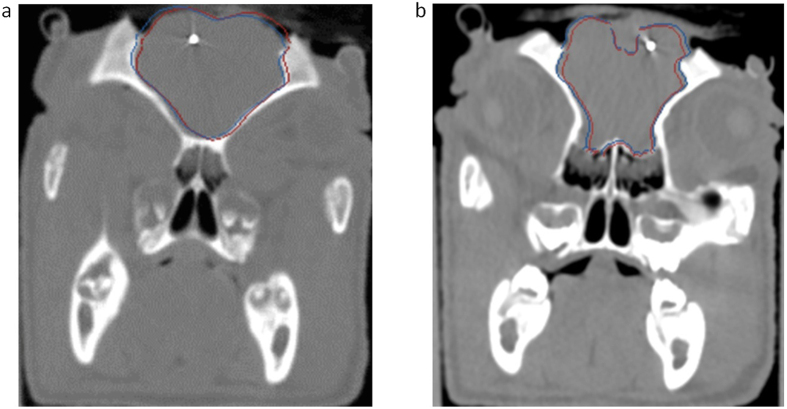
Comparisons of brain contours between the model-predicted image and the actual postretraction CT image (subject 1). (a) One slice of a preretraction image in the nonretraction area was overlaid with the corresponding brain contours of the model-predicted image (red) and the actual CT image (blue). (b) One slice in the retraction area. The brain contours of the model-predicted image and the brain edge of the actual CT image were identified separately and are shown as red lines and blue lines, respectively.
